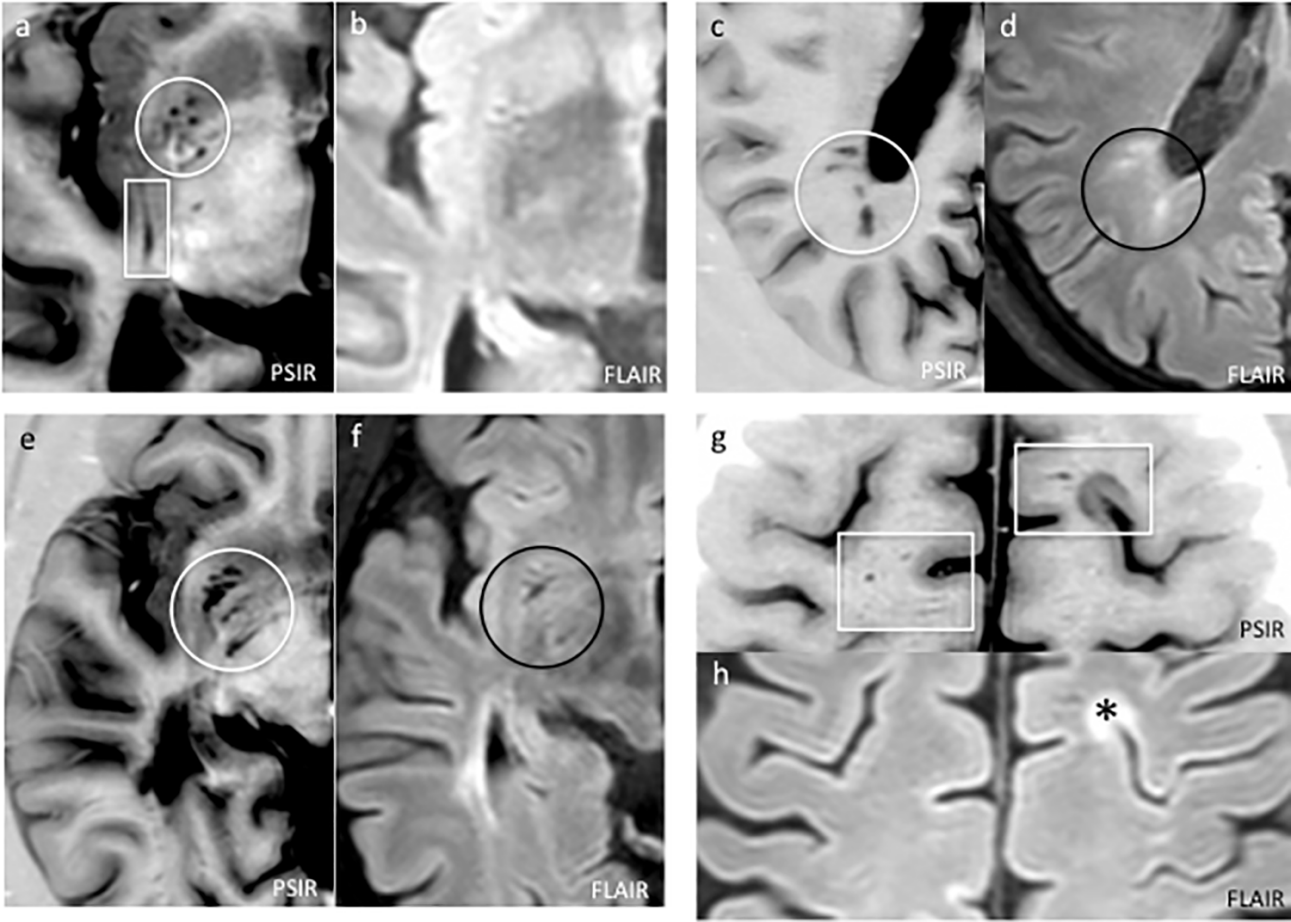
The comparison of PSIR (a,c,e,g) and FLAIR (b,d,f,h) images allows a clear discrimination of eVRS from white matter and grey matter lesions. In (a) and (b) several round-shaped (circle) and one stripe-like (rectangle) eVSR are clearly identified on PSIR, while on the FLAIR image they can hardly be recognised. A cluster of small inflammatory lesions that appear hypointense in PSIR and hyperintense in FLAIR are visible in (c) and (d). The comparison of PSIR and FLAIR images is necessary to discriminate small inflammatory lesions from eVRS. Several eVRS can be observed in the basal ganglia in (e) and (f): also in this case PSIR significantly improves the evaluation of their number and volume. In (g) and (f) some eVRS (rectangles) are observed in the convexity of a CIS patient (* indicates a mixed white/grey matter lesion).
